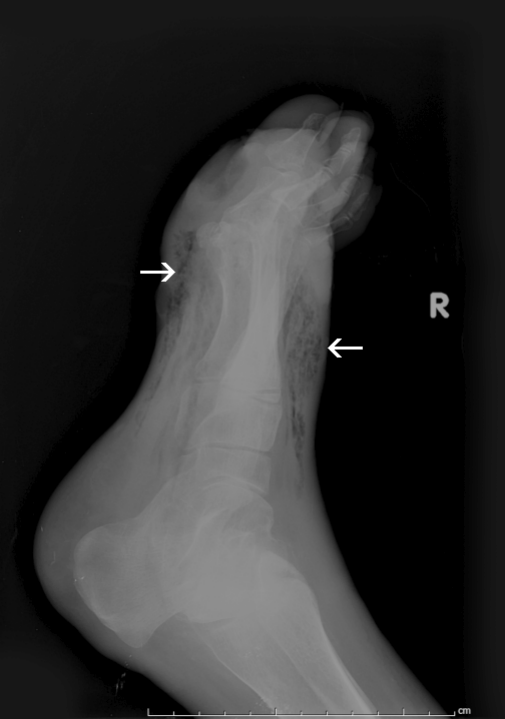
X-ray reveals soft-tissue gas consistent with gangrene.
